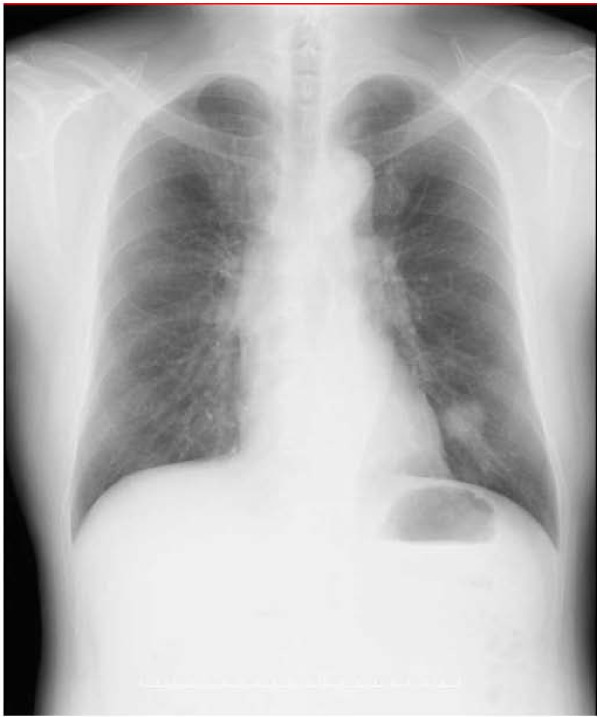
Chest radiography revealed a mass shadow in the left lower lung field.
